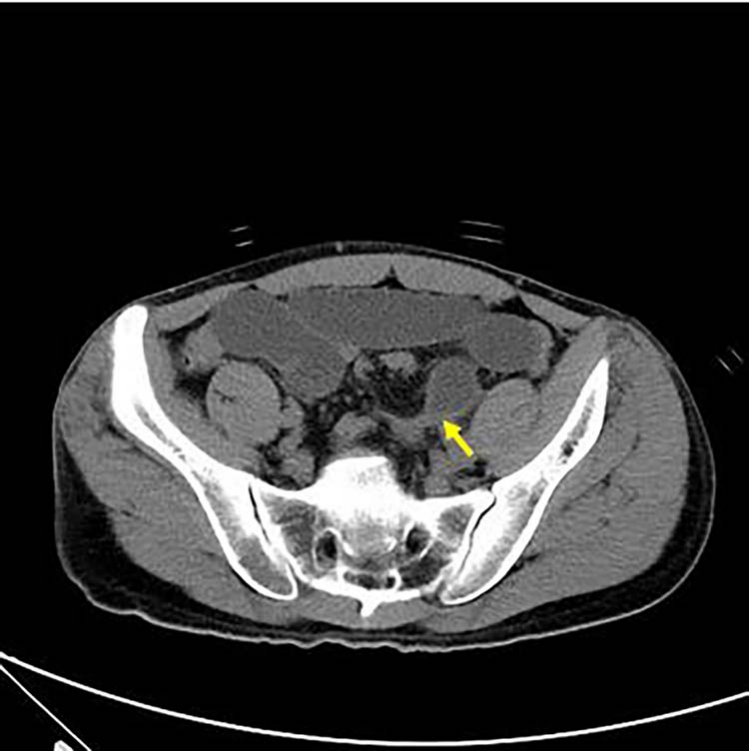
Axial view of abdominal CT revealing intersigmoid hernia with arrow indicating the leading point.
